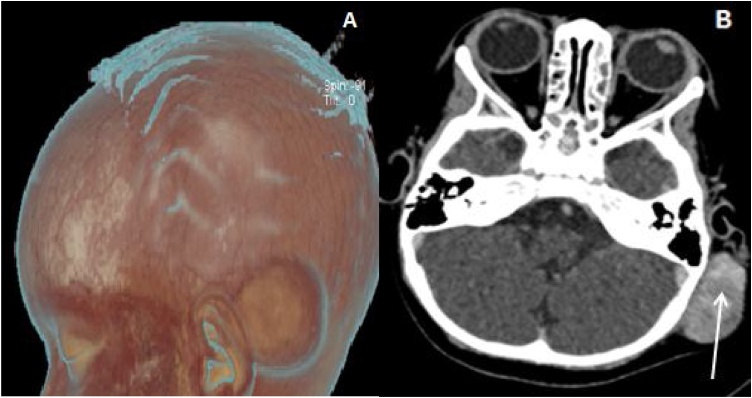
Patient #2, Radial reconstruction on CT-scan (A) and non-contrast CT-scan of head (B) showed a left retro auricular heterogeneous lesion (white arrow) measured at 41 mm as larger diameter, richly vascularized without bony involvement.
